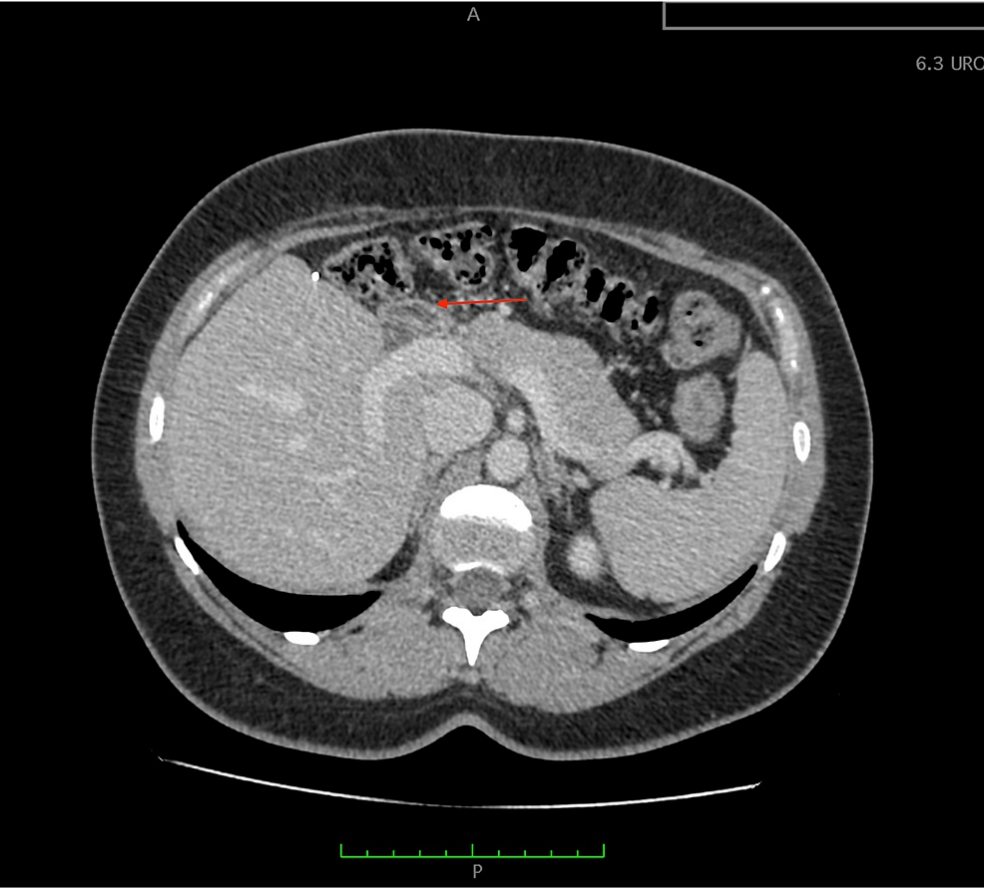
Follow-up CT scan five years after the surgery. Following resection, the postoperative anatomical location (red arrow).
